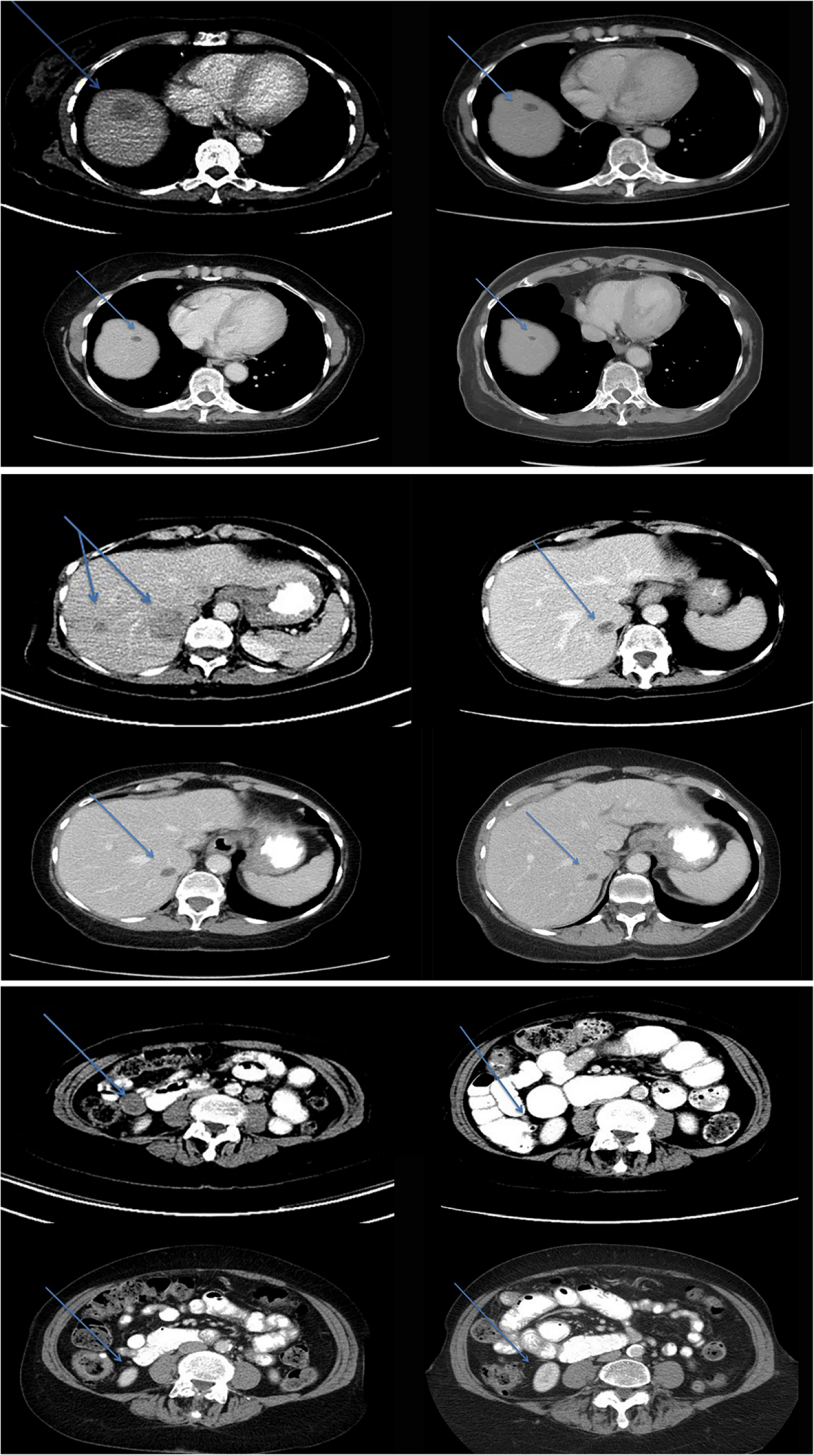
Top 4-up images, Patient #5, note the contrast enhanced, transverse abdominal computed tomography (CT) reveal a large solitary CMM metastasis on 2/2016, 7/2016, 4/2017 and 10/18 respectively (arrows). Middle 4-up CT-images reveal two additional CMM metastases on 2/2016, 7/2016, 2//2017 and 10/2018 respectively. Bottom 4-up CT images of a 2.7 mm abdominal implant prior to treatment on 2/2016, 7/2016, 4/2017 and 10/2018 where resolution is noted
